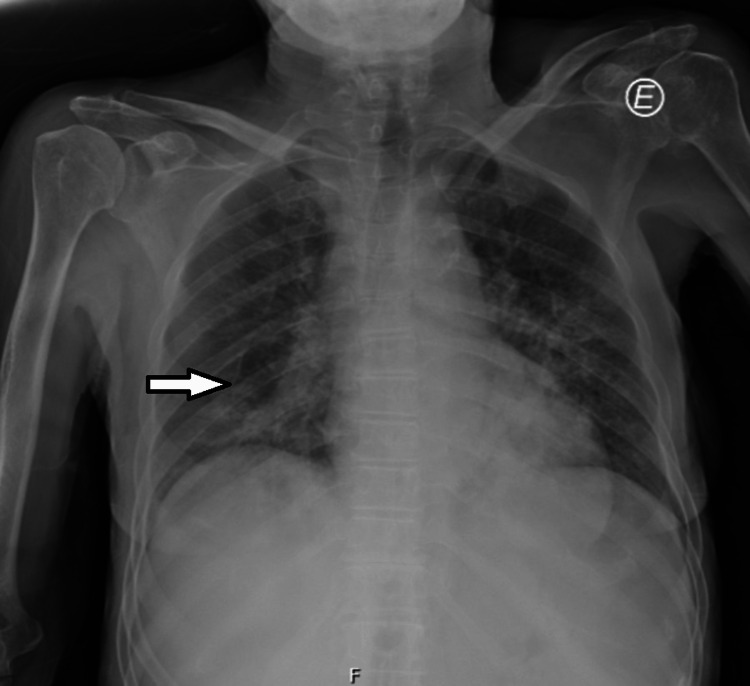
Chest radiograph at admission, showing a diffuse, bilateral interstitial infiltrates, and consolidation in the lower third of the right hemithorax
